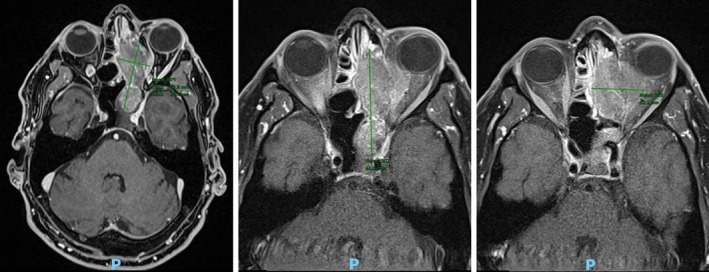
MRI orbit face neck imaging showing a large locally advanced left‐sided sinonasal mass involving the left ethmoid, left sphenoid, and left superior nasal cavity with gross invasion of the left medial orbit where there is a left optic nerve compression. There is also evidence of invasion of the anterior skull base with suspicious early dural invasion. The sphenoid component of the tumor is bordering the left precavernous/cavernous internal carotid artery and left optic canal without invasion.
